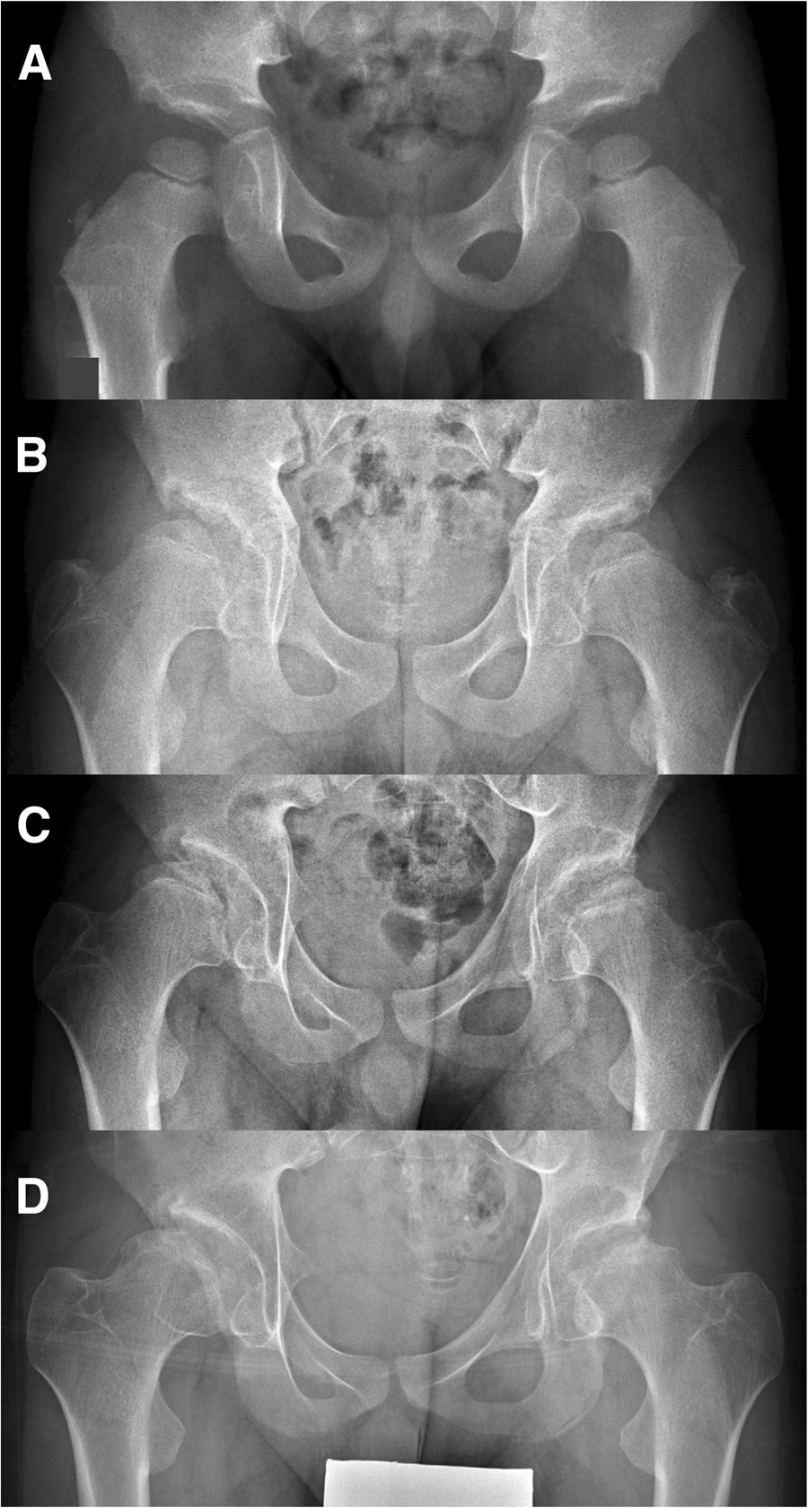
Aberrant hip development in a COMP-MED patient. A: At 3 years of age, the radiograph shows small and round femoral heads and irregular acetabular roof. Through the puberty and adolescence (B: At 11 years. C: At 12 years. D: At 13 years), the hips become dysplastic, uncovered and of flattened and irregular femoral heads.
